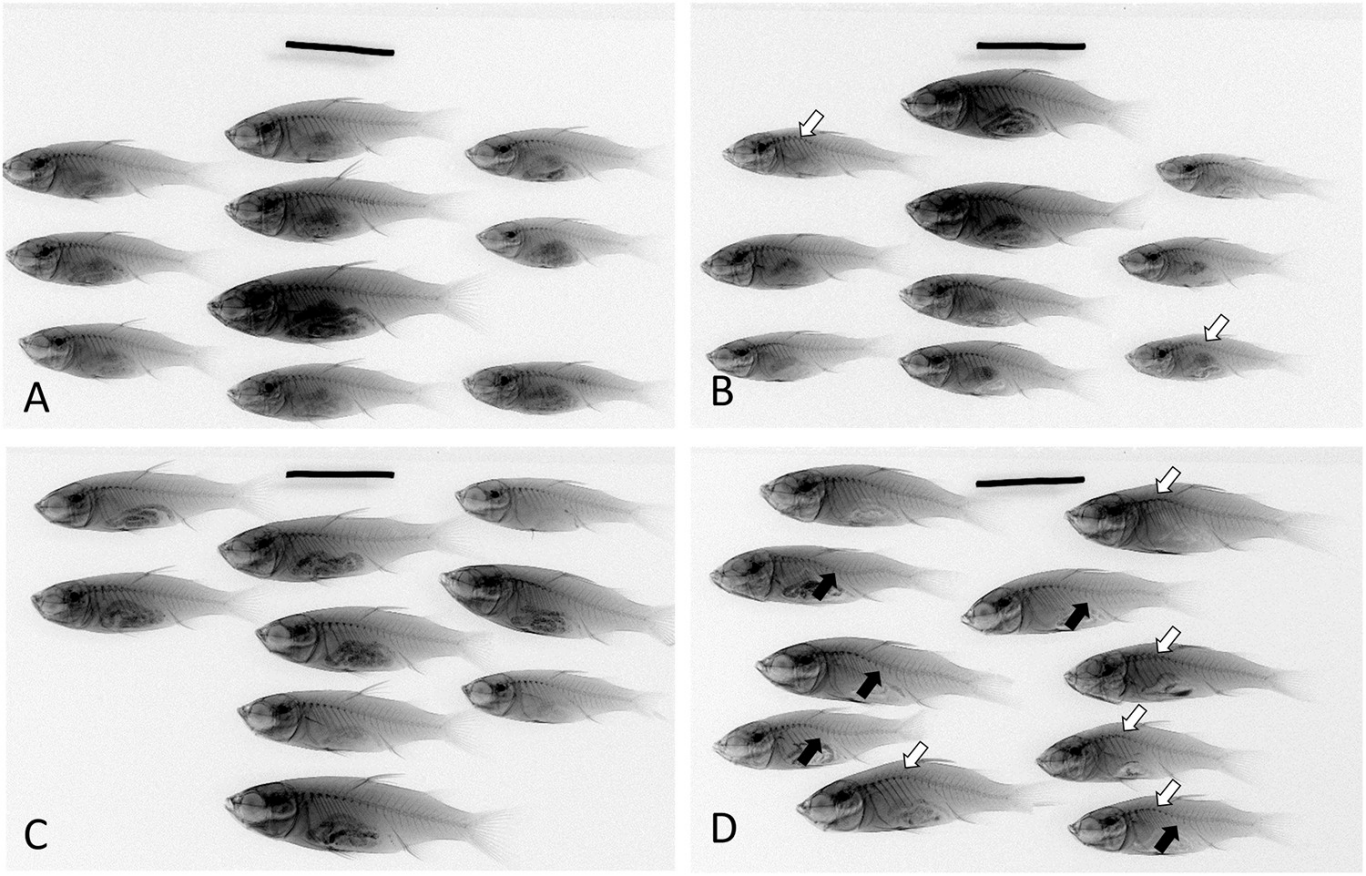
Lateral X-ray views of largescale mullet in Experiment 1. Fish were maintained for (A) 2 weeks at 26°C, (B) 2 weeks at 36°C, (C) 4 weeks at 26°C, or (D) 4 weeks at 36°C prior to X-ray. Open arrows indicate kyphosis, and solid arrows indicate lordosis. Scale bar, 10 mm.
